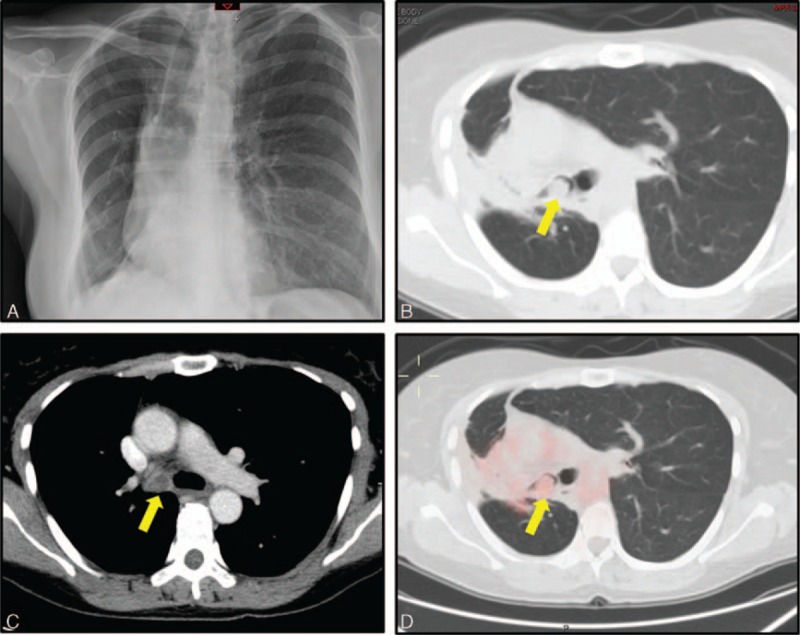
Radiological evaluation: chest x-ray (A) revealed a middle and lower pulmonary lobe collapse; contrast-enhanced CT-scan (B, C) showed the presence of a highly vascularized and round-shape solid lesion causing the subtotal obstruction of the main right bronchus; at ^18^F-FDG PET/CT scan (D) the lesion presented with a mild activity (SUVmax = 2.1), whereas no uptake was found in other areas. CT = computed tomography, ^18^F-FDG PET/CT = 18-fluoro-2-deoxy-d-glucose positron emission tomography/computed tomography.
